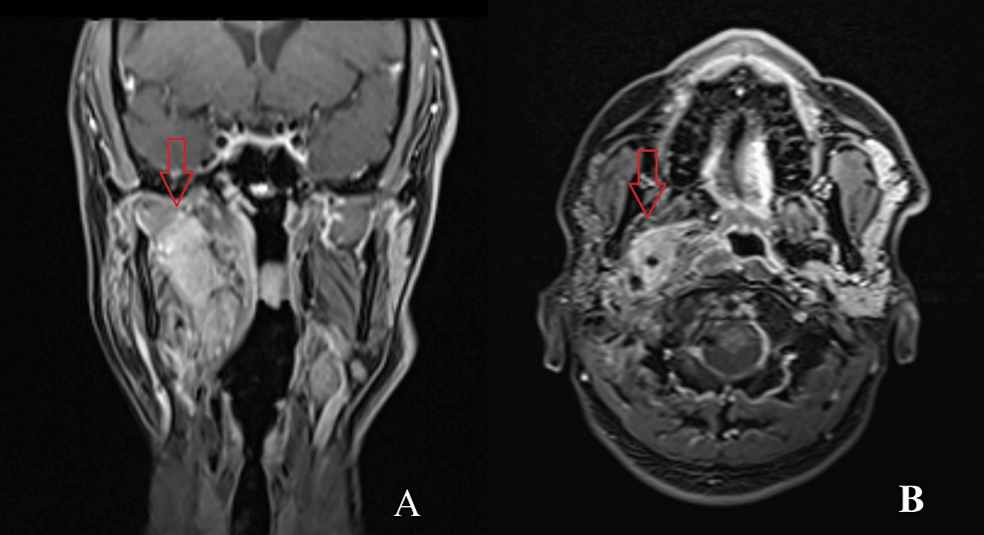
Magnetic resonance imaging showing posttreatment images of local tumor control eight years after treatment Red arrows show the treated disease site. (A) Coronal view. (B) Axial view.
